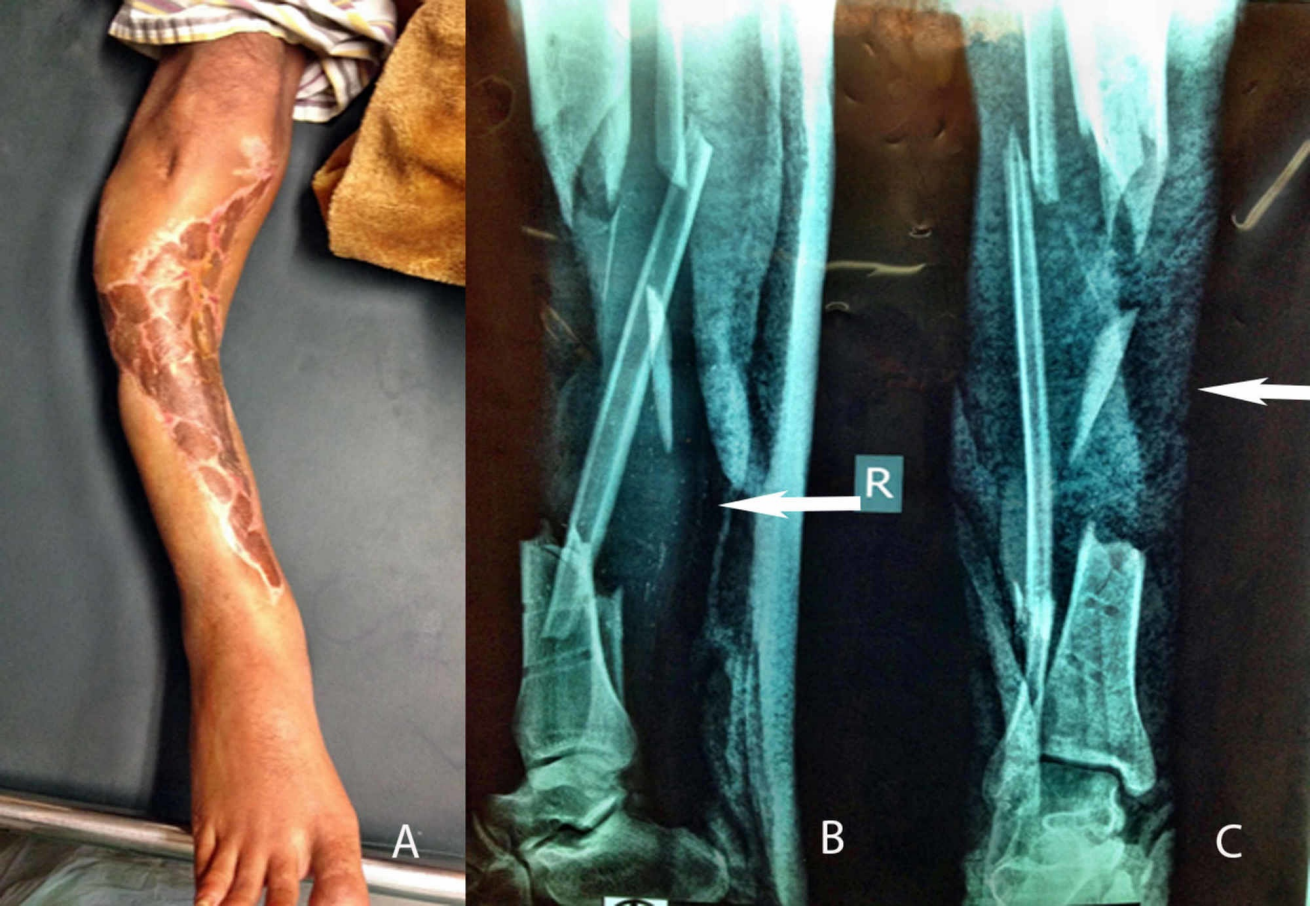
Preoperative pictures of post-traumatic bone defect with a live view (1A), lateral radiographic view (1B), and anteroposterior radiographic view (1C)
